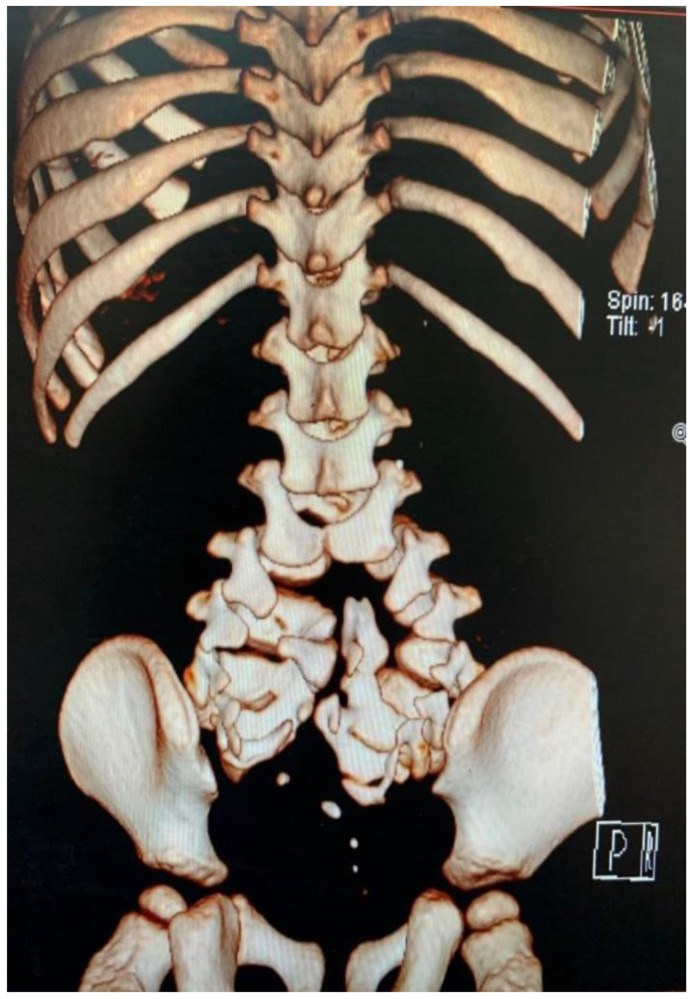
Three-dimensional reconstruction of computed tomography of the spine from mid-thoracic region to sacrum reveals duplication of the vertebral column from lumbar vertebrae 3 to the coccyx.
